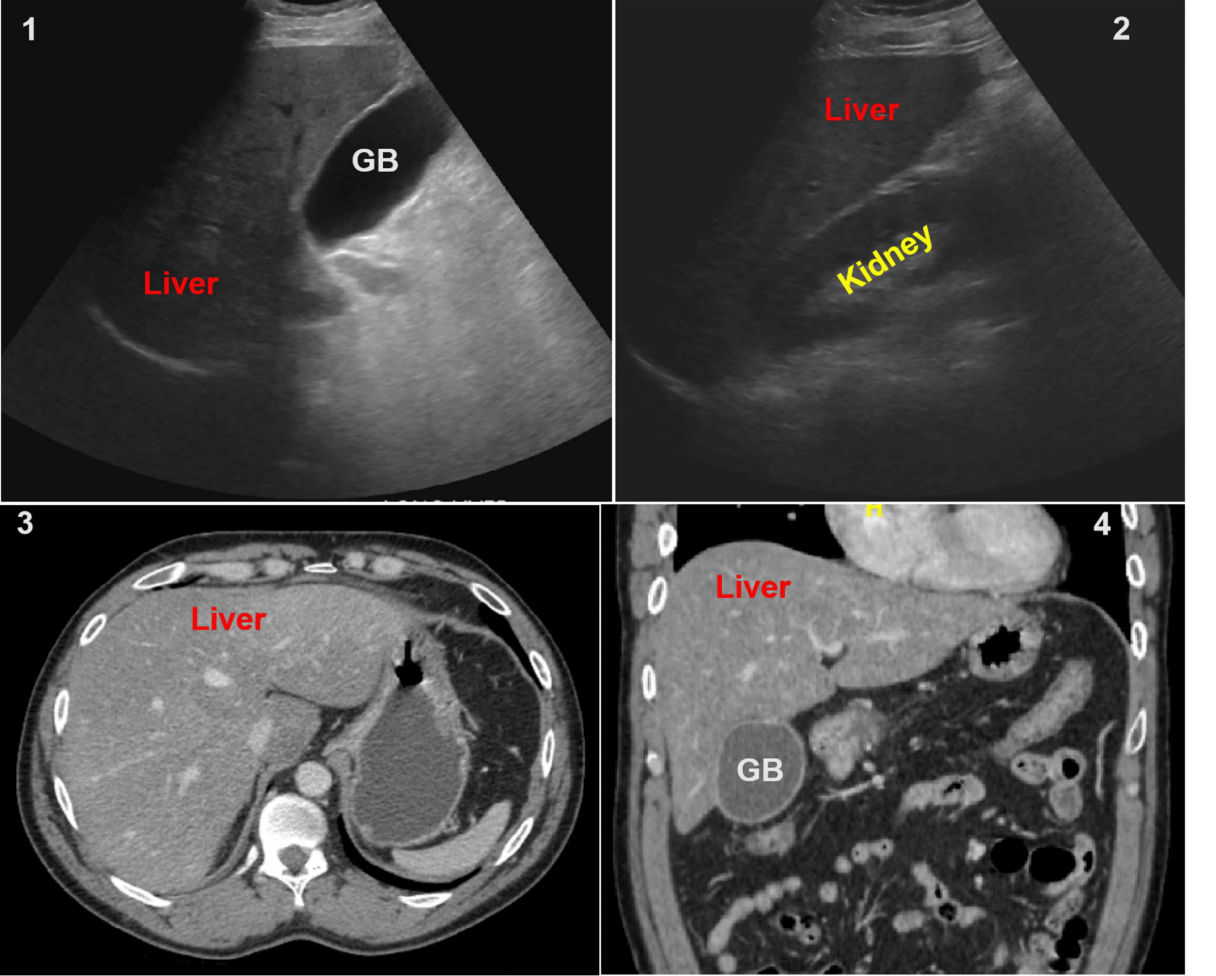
Ultrasound and computed tomography images Ultrasound images (A, B) revealed a hyperechoic liver and a distended gallbladder (GB) with borderline wall thickness, but without cholelithiasis. Computed tomography (C, D) revealed diffuse low-attenuation of the liver compatible with fatty infiltration and a distended GB.
